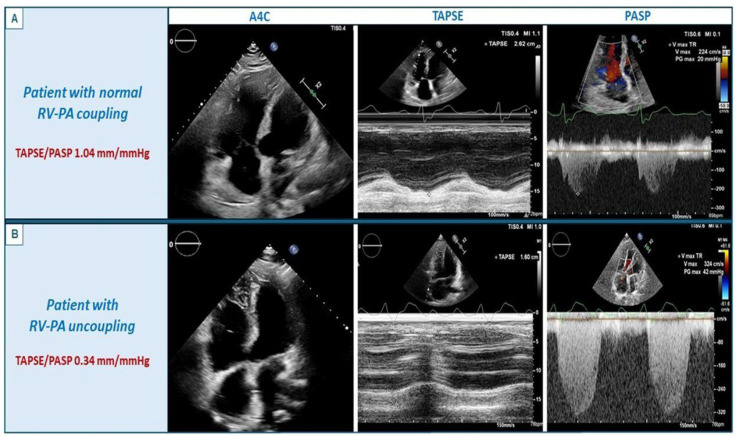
Panel (A): Echocardiographic images of a patient with repaired tetralogy of Fallot and normal RV-PA coupling (TAPSE/PASP 0.93). Panel (B): Echocardiographic images of a patient with repaired tetralogy of Fallot and RV-PA uncoupling (TAPSE/PASP 0.31). Abbreviations: A4C: apical four chamber view; PASP: pulmonary artery systolic pressure; RV-PA: right ventricle–pulmonary artery; TAPSE: tricuspid annular plane systolic excursion.
